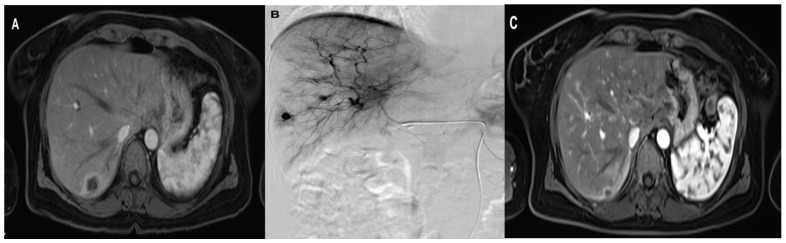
A 47-year-old woman with solitary liver metastasis in the right liver lobe treated with three sessions of Lipiodol + DSM TACE with Gemcitabine, Cisplatin, and Mitomycin. (A) pre-treatment axial MRI (B) During TACE (C) post-treatment axial contrast-enhanced MRI shows the significant downsizing of the metastasis.
